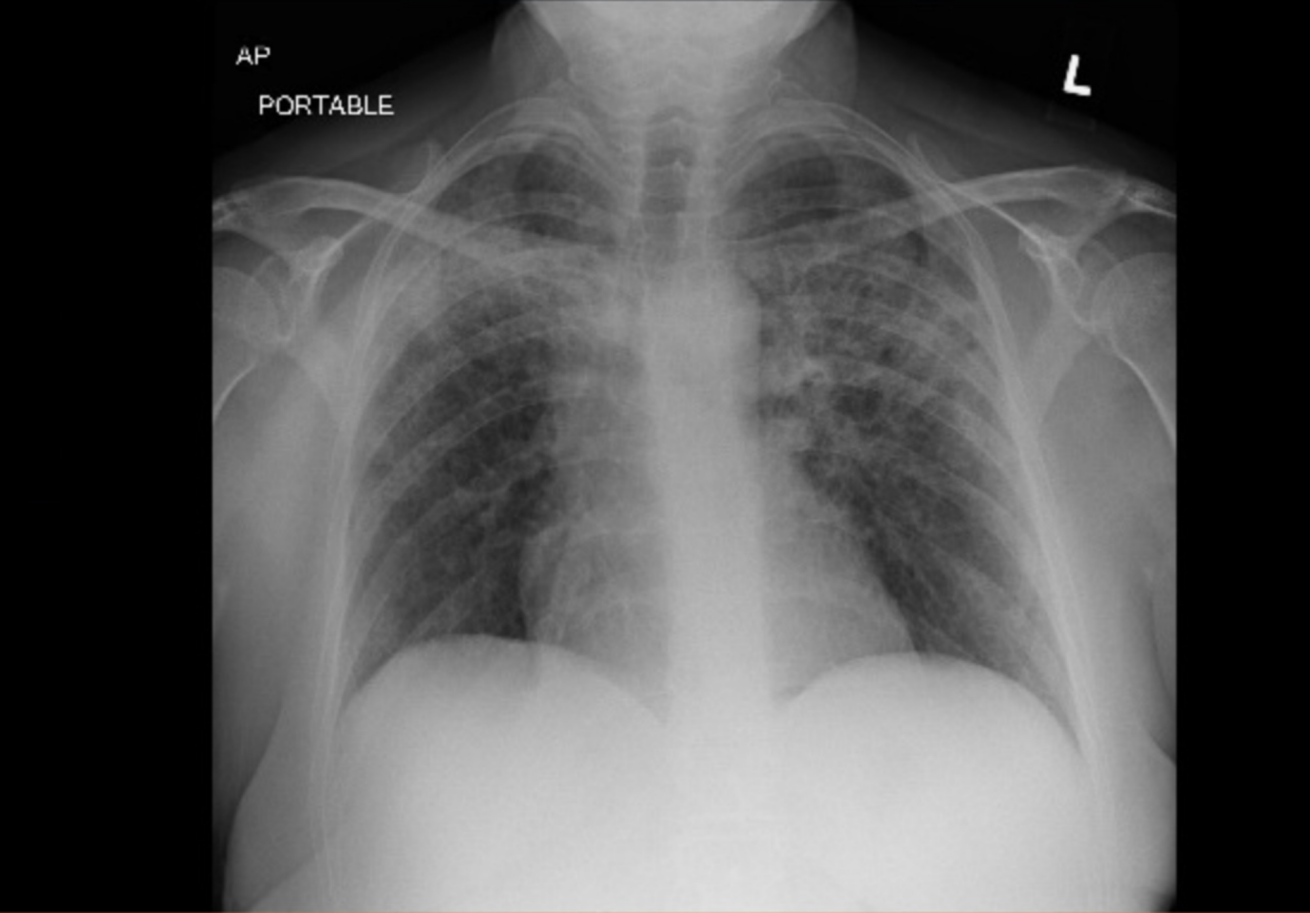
Anterior-posterior chest X-ray showing interval improvement of bilateral airspace opacities on discharge from the intensive care unit
